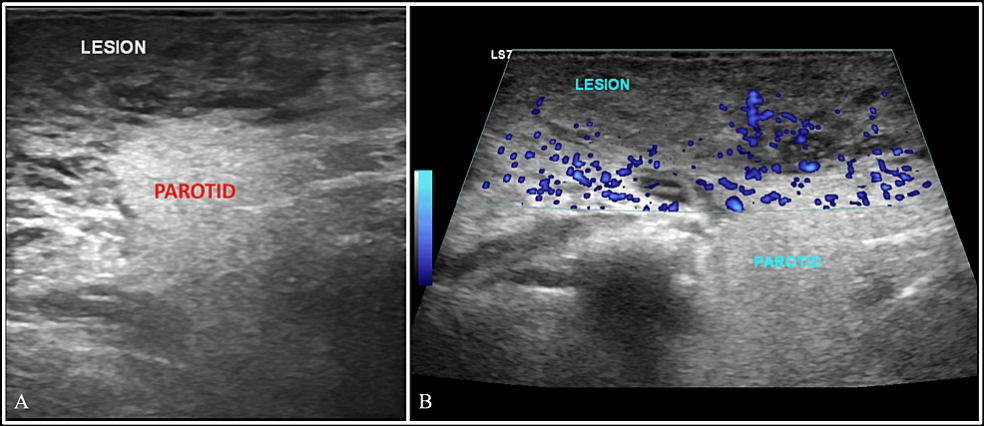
Greyscale ultrasound of the neck Greyscale ultrasound (A) and power doppler (B) images of the neck at the infraauricular level on the right side, showing a large heteroechoic mass superficial to the parotid gland, showing few areas of vascularity within it. Note the difference in the echotexture between the soft tissue mass and the parotid.
